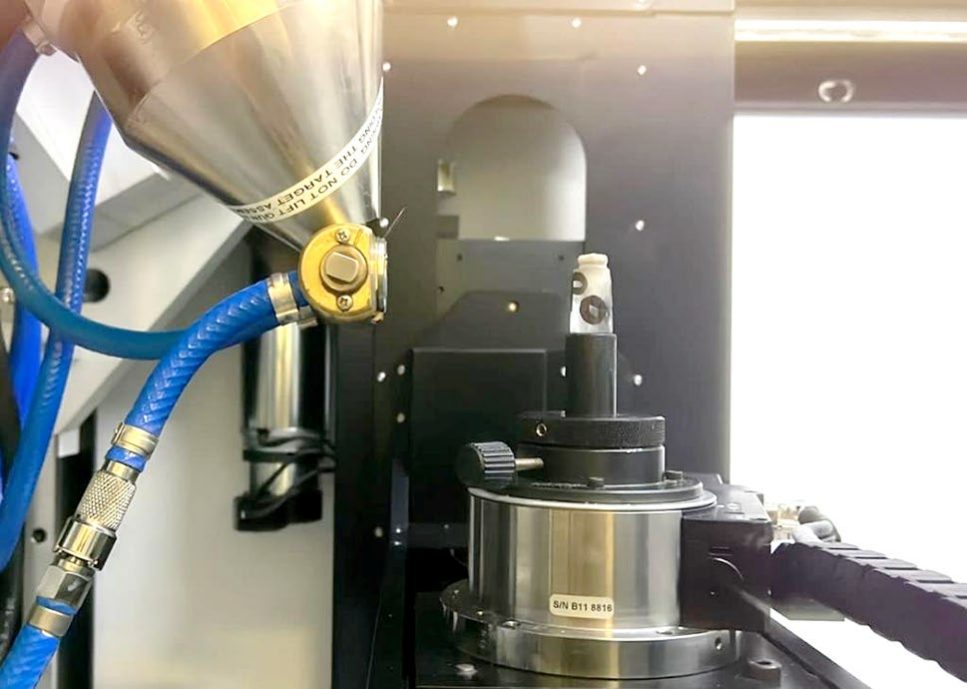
mounted sample for CBCT measurement
